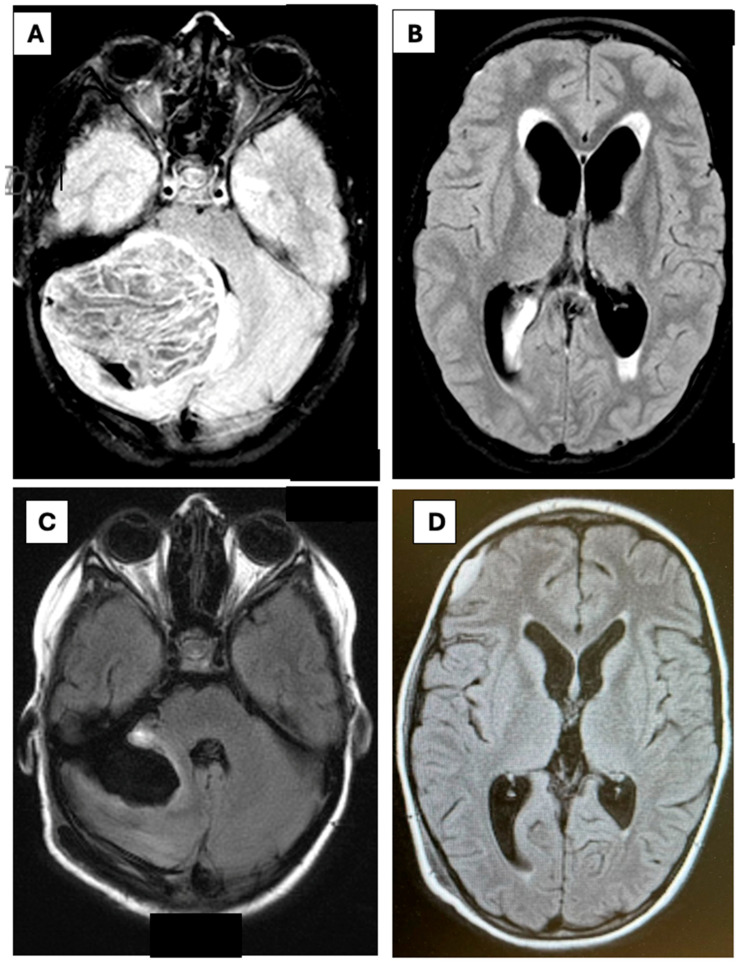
(A) Axial T2-weighted fluid-attenuated inversion recovery (T2-FLAIR) MRI demonstrating a cerebellar dysplastic gangliocytoma in a 10-year-old patient diagnosed with Lhermitte–Duclos Syndrome. (B) The same axial T2-FLAIR sequence reveals hydrocephalic enlargement of the ventricular system. (C) A follow-up rapid MRI using T2-FLAIR fluid-attenuated inversion recovery was performed 48 h after external ventricular drain (EVD) closure, prior to removal. Imaging shows re-expansion of the fourth ventricle to normal dimensions, indicating resolution of the mass effect previously exerted by the tumor. (D) Postoperative rapid MRI using T2-FLAIR imaging demonstrates reduced ventricular size and cerebrospinal fluid diapedesis, consistent with resolving hydrocephalus.
